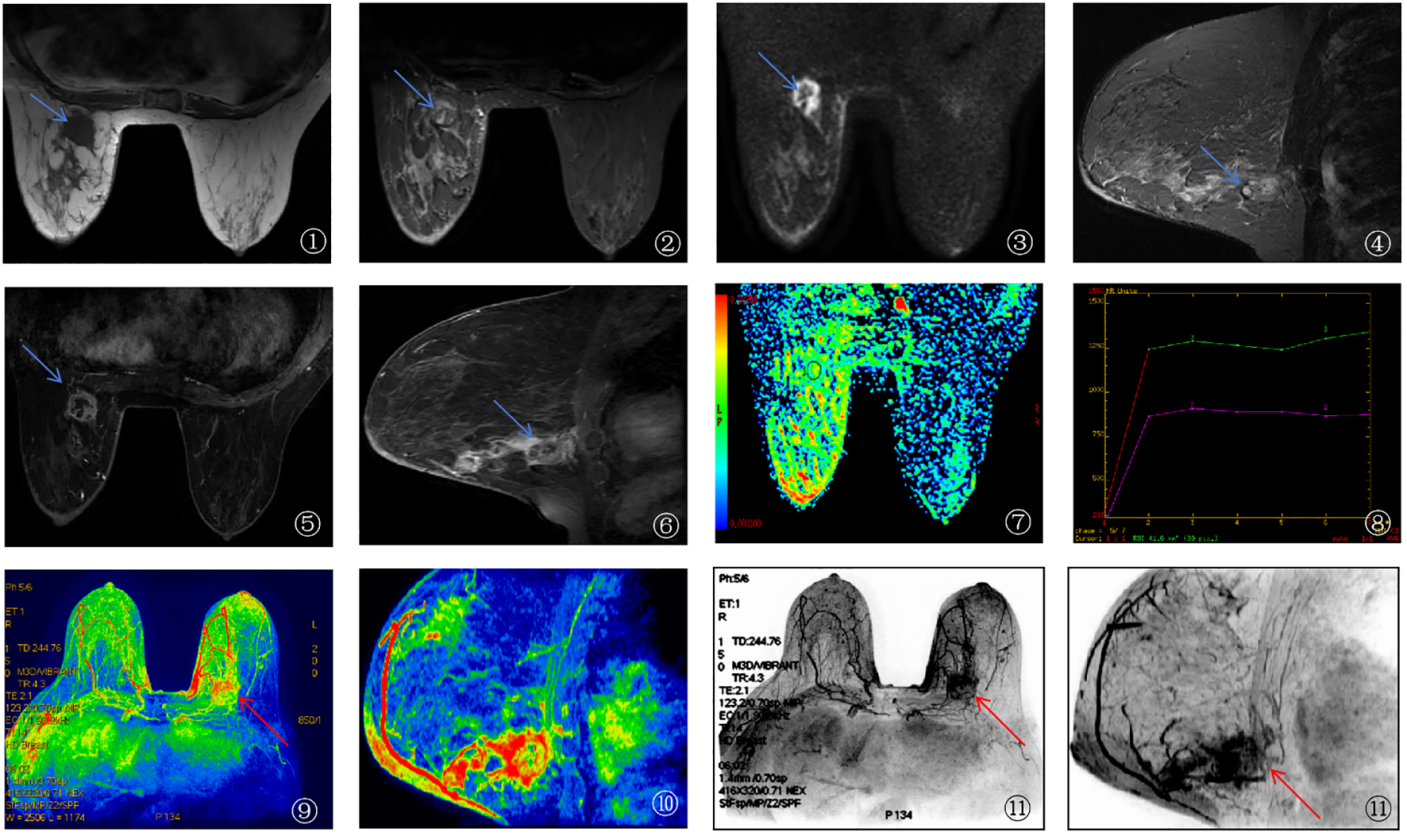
3.0T HR-MRI imaging findings of breast cancer patients.
